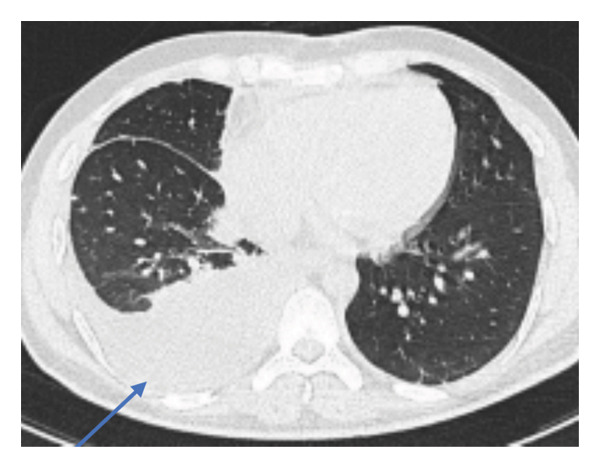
An axial view of HRCT demonstrated moderate right pleural effusion (blue arrow). (HRCT: high‐resolution computed tomography).
